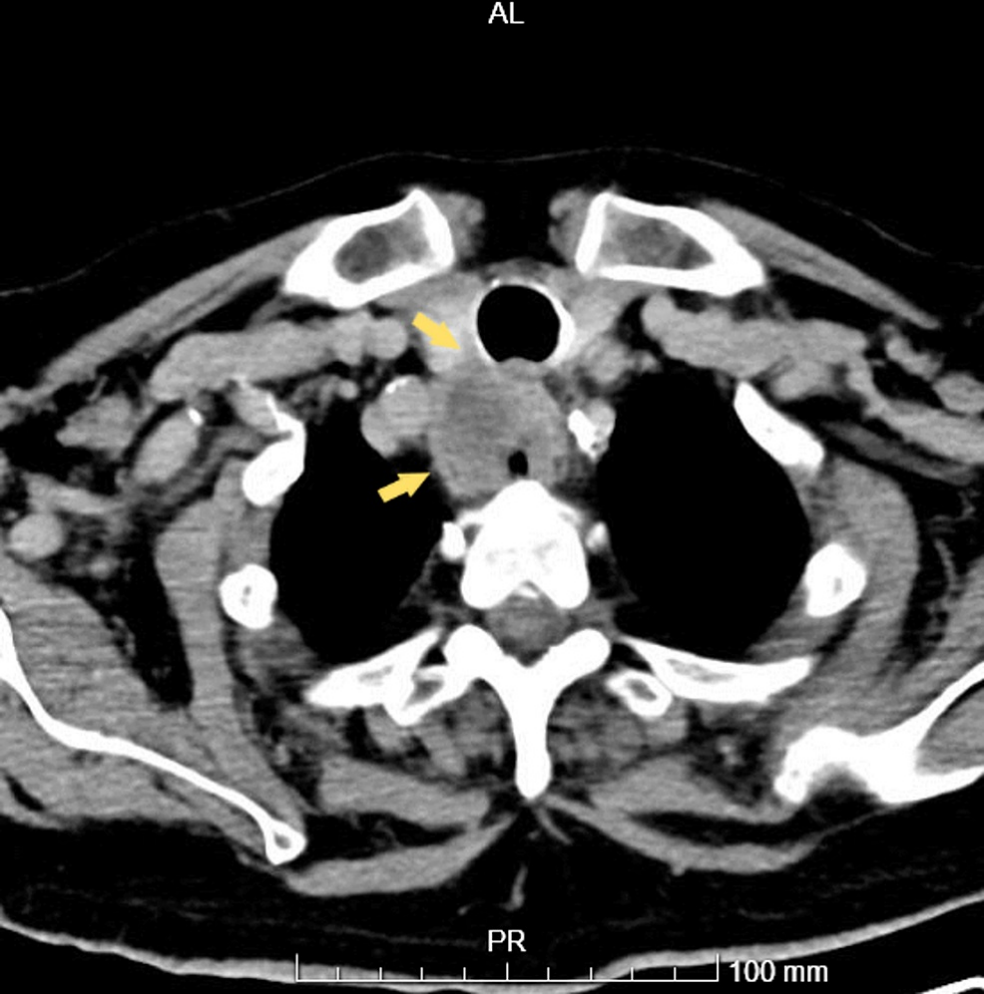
Thoracic CT: Esophageal mass merged with the trachea.
